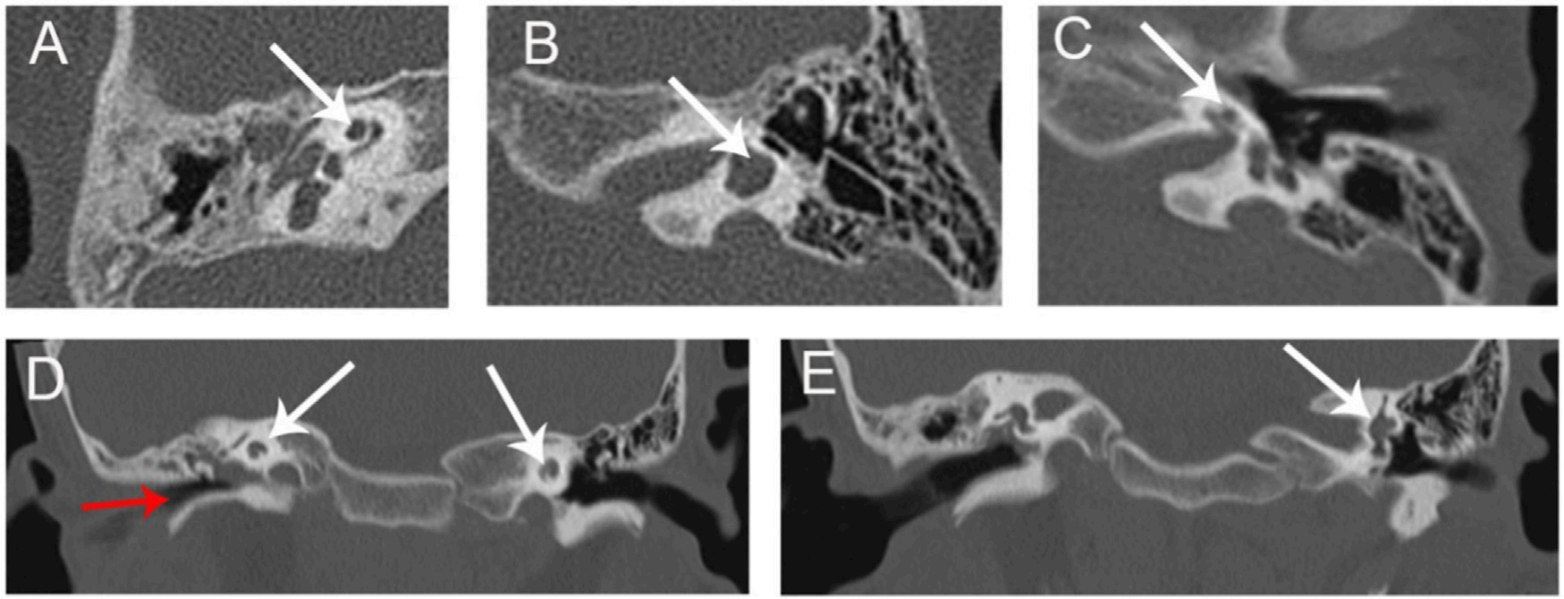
HRCT of proband (A) Cochlear hypoplasia in the right ear (arrow). (B) Lateral semicircular canal-vestibule dysplasia in the left ear (arrow). (C) Cochlear hypoplasia with less than two turns in the left ear. (D) Soft tissue shadow in the right middle ear (red arrow). Cochlear hypoplasia on both sides (white arrows). (E) Lateral semicircular canal-vestibule dysplasia in the left ear (arrow). (A–C) Coronal view; (D,E) Axial view. HRCT, high-resolution computed tomography.
